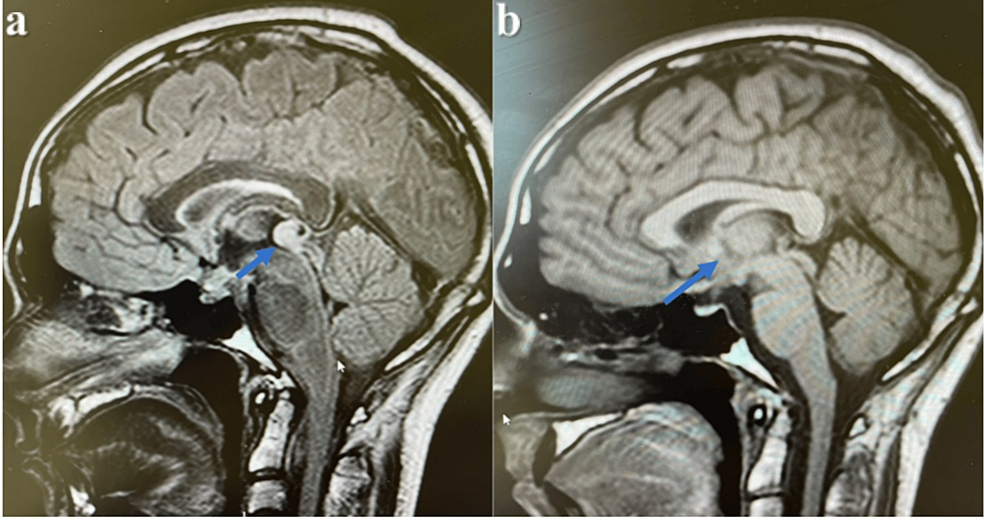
MRI brain diagnostic imaging reveals a prominent pineal region mass, and (b) Notable pituitary stalk thickening
